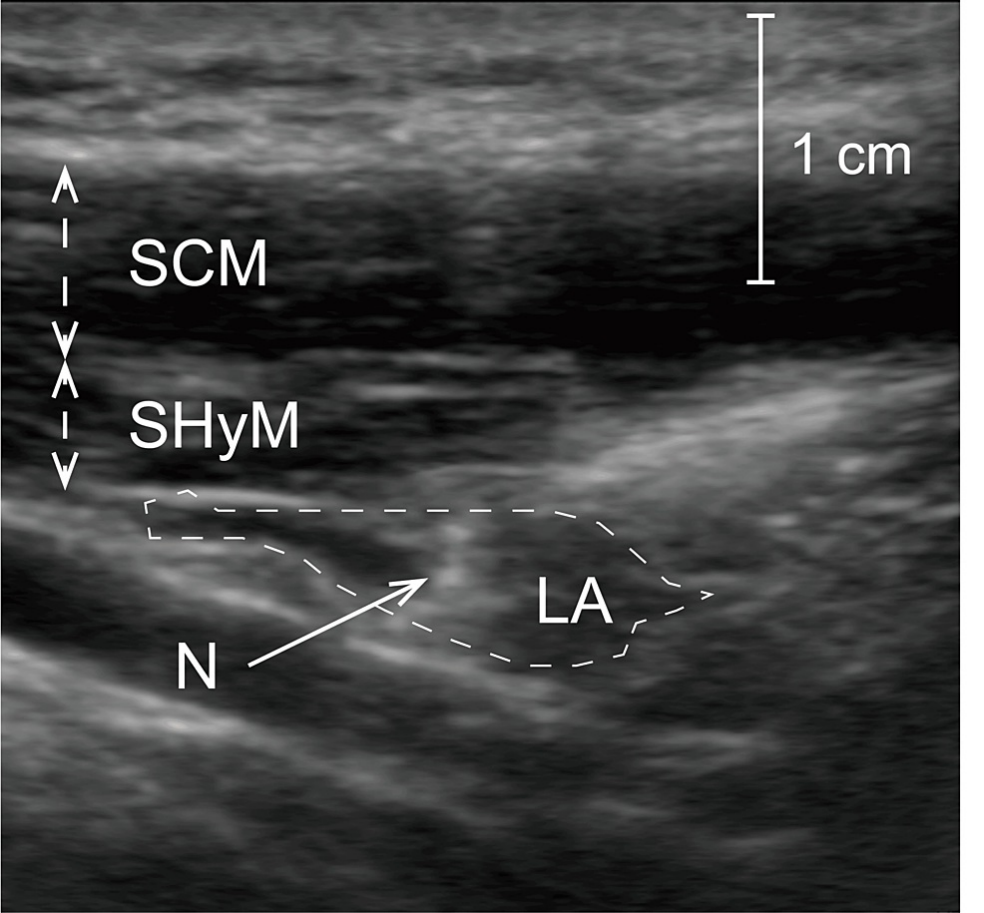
Ultrasound images of an ultrasound-guided selective glossopharyngeal nerve block The sternocleidomastoid muscle and stylohyoid muscle can be observed. We confirmed the spread of the local anesthetic. LA, local anesthetic; N, needle; SCM, sternocleidomastoid muscle; SHyM, stylohyoid muscle
